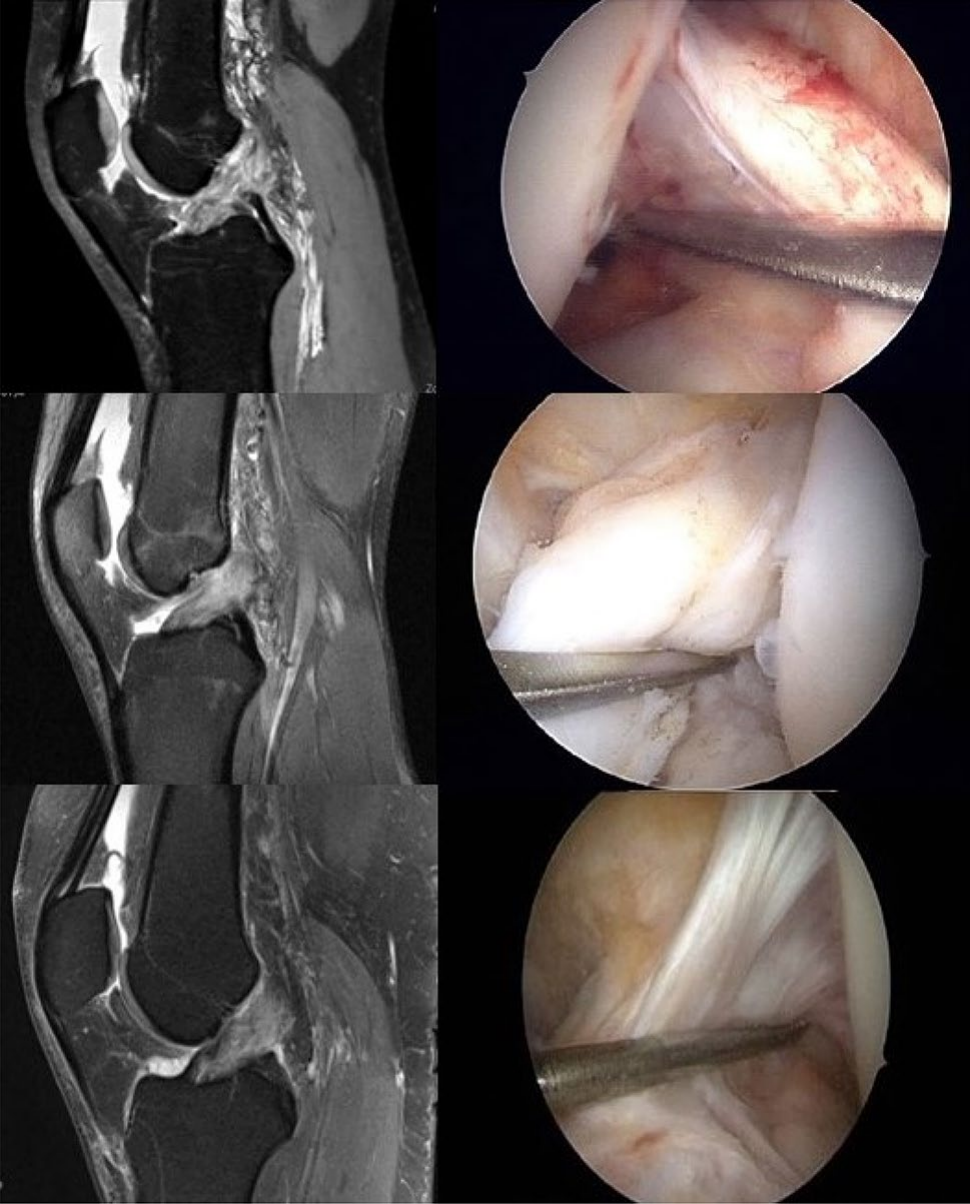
MR imaging after ACL injury and arthroscopic view of healed ACL 6 weeks after injury
